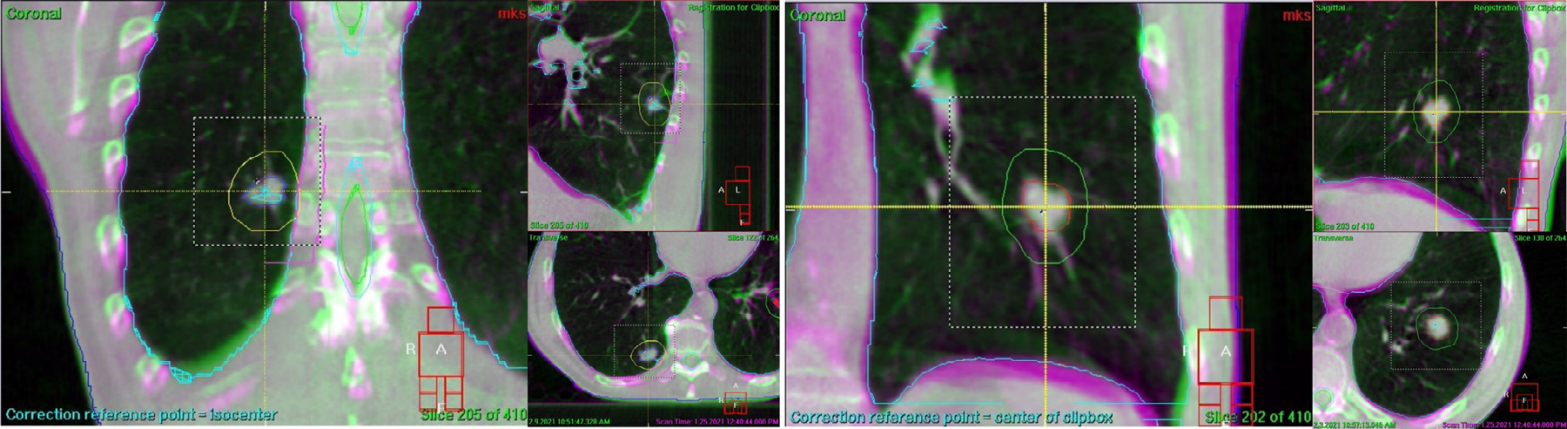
Deep inspiration breath hold–only cone beam computed tomography (CBCT) scans of both targets (pink = planning computed tomography; green = CBCT). CBCT with repeated deep inspiration breath hold has almost a diagnostic quality in tissues with high contrast and makes daily matching more precise than 4-dimensional computed tomography.
